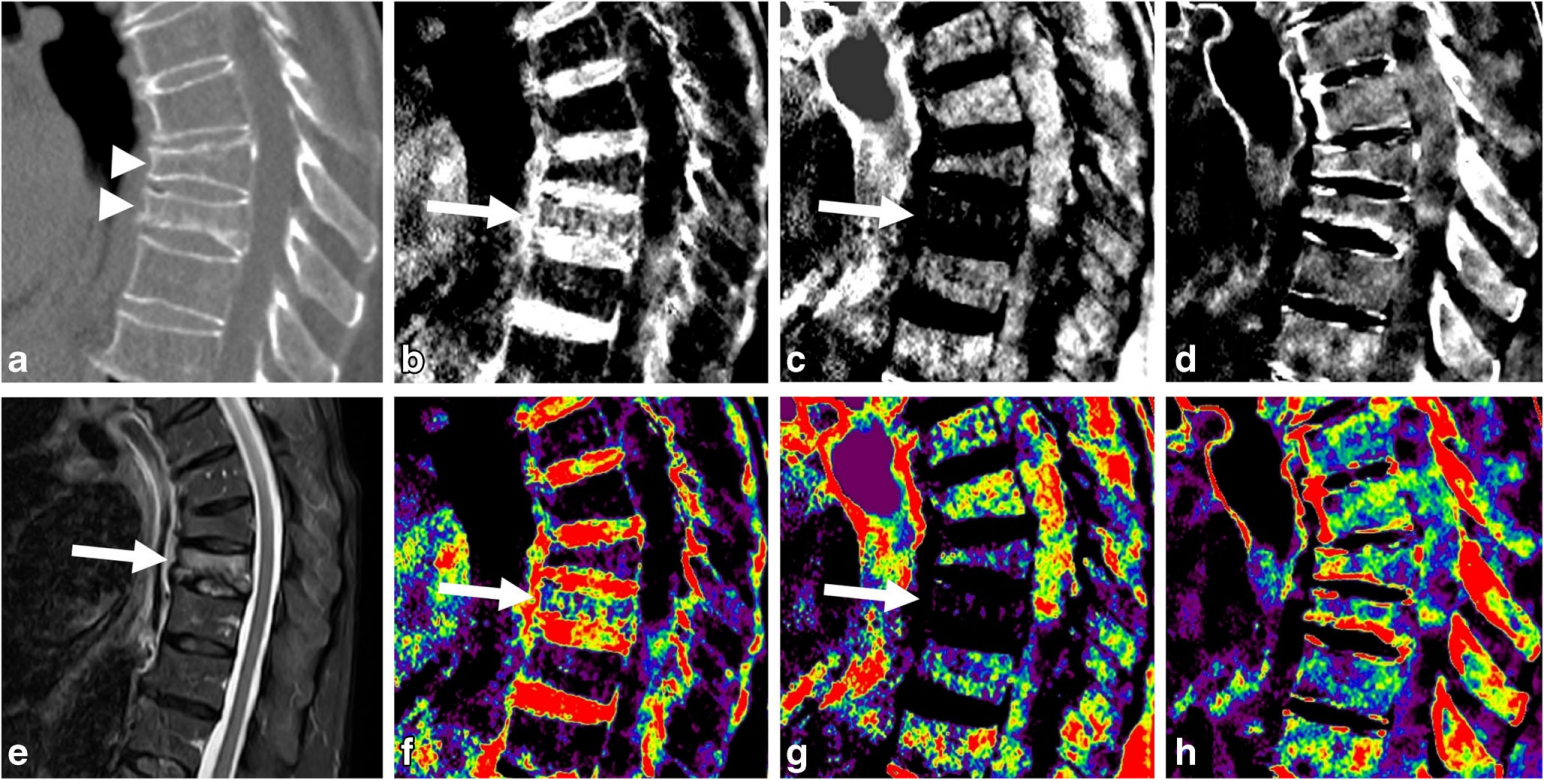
CT and MR images of a 67-year-old female patient admitted for acute back pain and history of osteopenia. Sagittal reformation equivalent to a conventional CT examination (a) reveals compression fractures of Th10 and 11 (*arrowheads*). Th11 presents a global increase in HU numbers possibly due to condensation, but no definite signs of an acute fracture are seen. Material-specific density maps for water (b), fat (c), and hydroxyapatite (d), and respective color-coded overlays (f, g, h) reveal an increase in water-specific density in the red-marrow map (b and f;* arrow*) and a decrease in fat-specific density in the yellow-marrow map (c and g;* arrow*) in Th11 compared to adjacent vertebral bodies, including Th10. The hydroxyapatite-specific map suggests a density increase in the lower endplate in Th11 (d and h). The sagittal fluid-sensitive fat-saturated STIR sequence from the subsequent MR examination confirms the presence of an edema-equivalent signal alteration in Th11, thus confirming an acute fracture (*arrow*), but not in Th10, thus confirming a chronic fracture (e)
